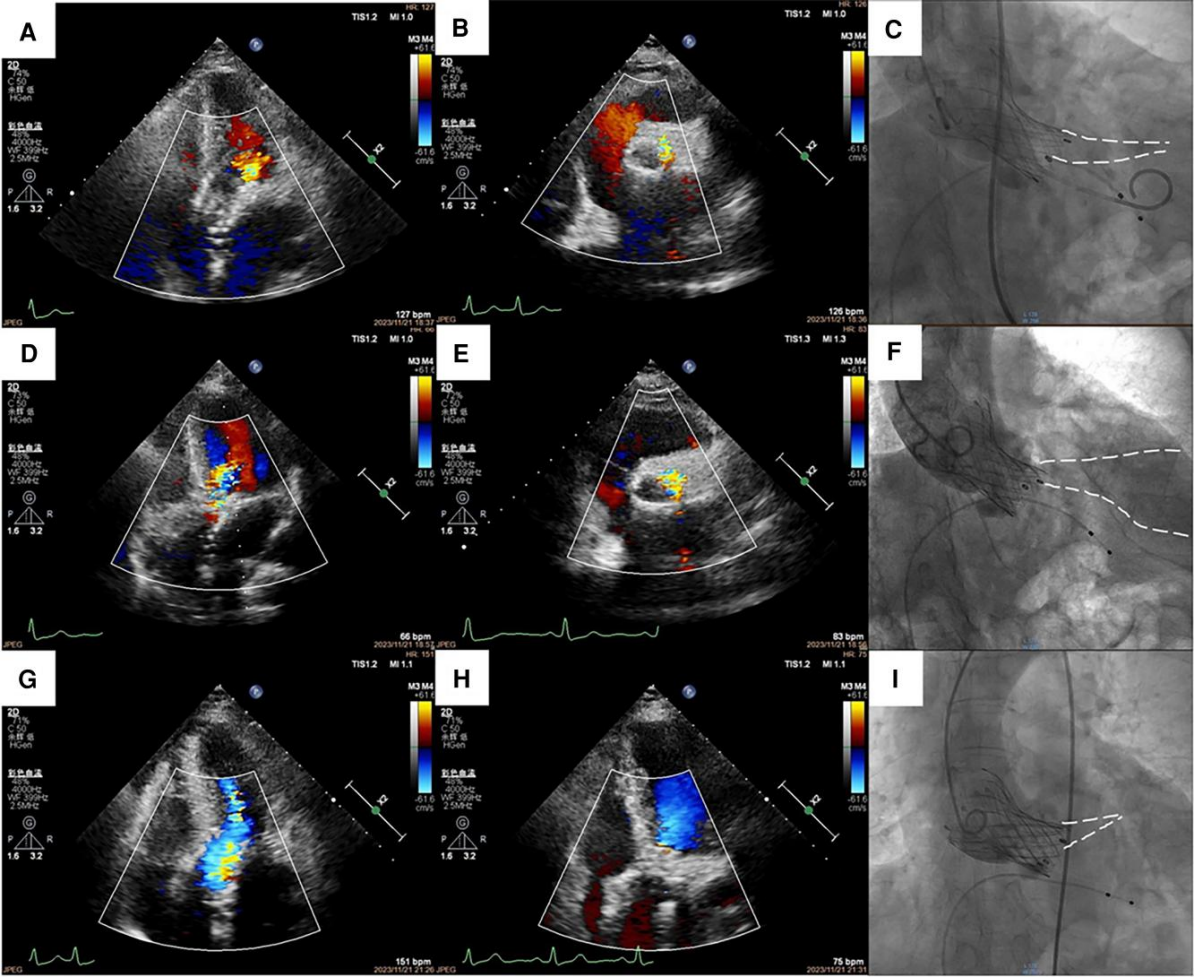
Intra-operative evaluation of aortic paravalvular regurgitation using transthoracic echocardiography and aortography. Patient with atrial tachyarrhythmias, apical five-chamber view (*A*; Supplementary material online, *Video S1*) and parasternal short-axis view (*B*; Supplementary material online, *Video S2*) on transthoracic echocardiography and cusp-overlap view on aortography (*C*; Supplementary material online, *Video S3*) showing mild paravalvular regurgitation after first prosthesis implantation. After the patient restoring normal haemodynamics, apical five-chamber view (*D*; Supplementary material online, *Video S4*) and parasternal short-axis view (*E*; Supplementary material online, *Video S5*) on transthoracic echocardiography and cusp-overlap view on aortography (*F*; Supplementary material online, *Video S6*) showing moderate-to-severe paravalvular regurgitation. After valve-in-valve procedure, apical five-chamber view (*G*; Supplementary material online, *Video S7*) showing trace paravalvular regurgitation while atrial tachyarrhythmia reoccurring. After achieving normal rhythm and heart rate using propafenone, apical five-chamber view (*H*; Supplementary material online, *Video S8*) on transthoracic echocardiography and cusp-overlap view (*I*; Supplementary material online, *Video S9*) on aortography confirming trace paravalvular regurgitation. White dash line in *C*, *F*, and *I* indicated the border of paravalvular regurgitation on aortography.
